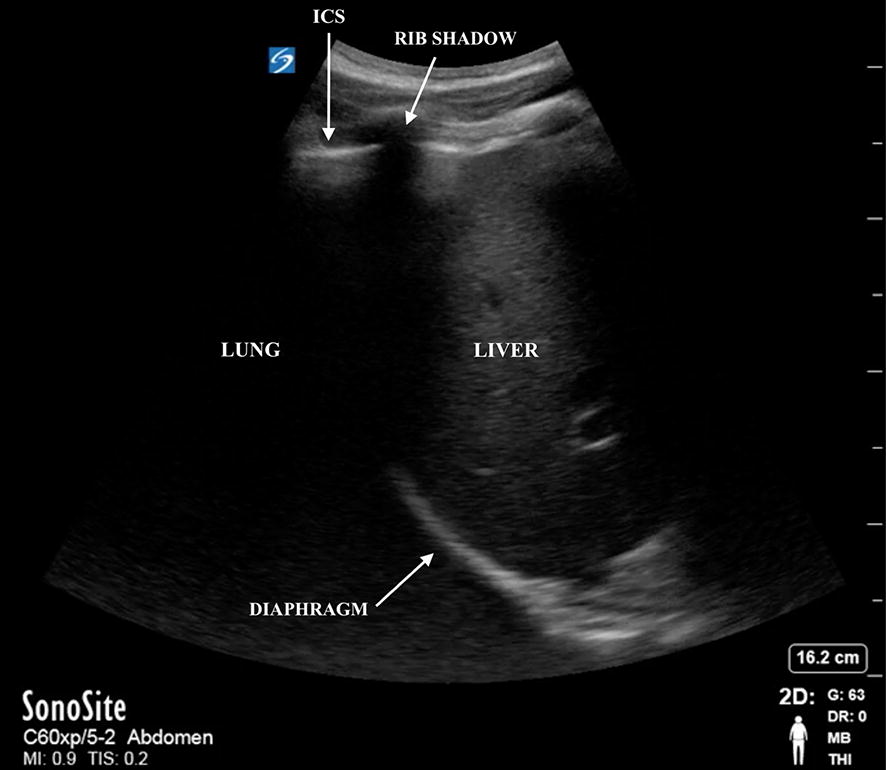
This is a right upper quadrant ultrasound image at the costophrenic angle demonstrating the starting point of the ultrasound-guided identification of the diaphragm, ribs, and intercostal space
